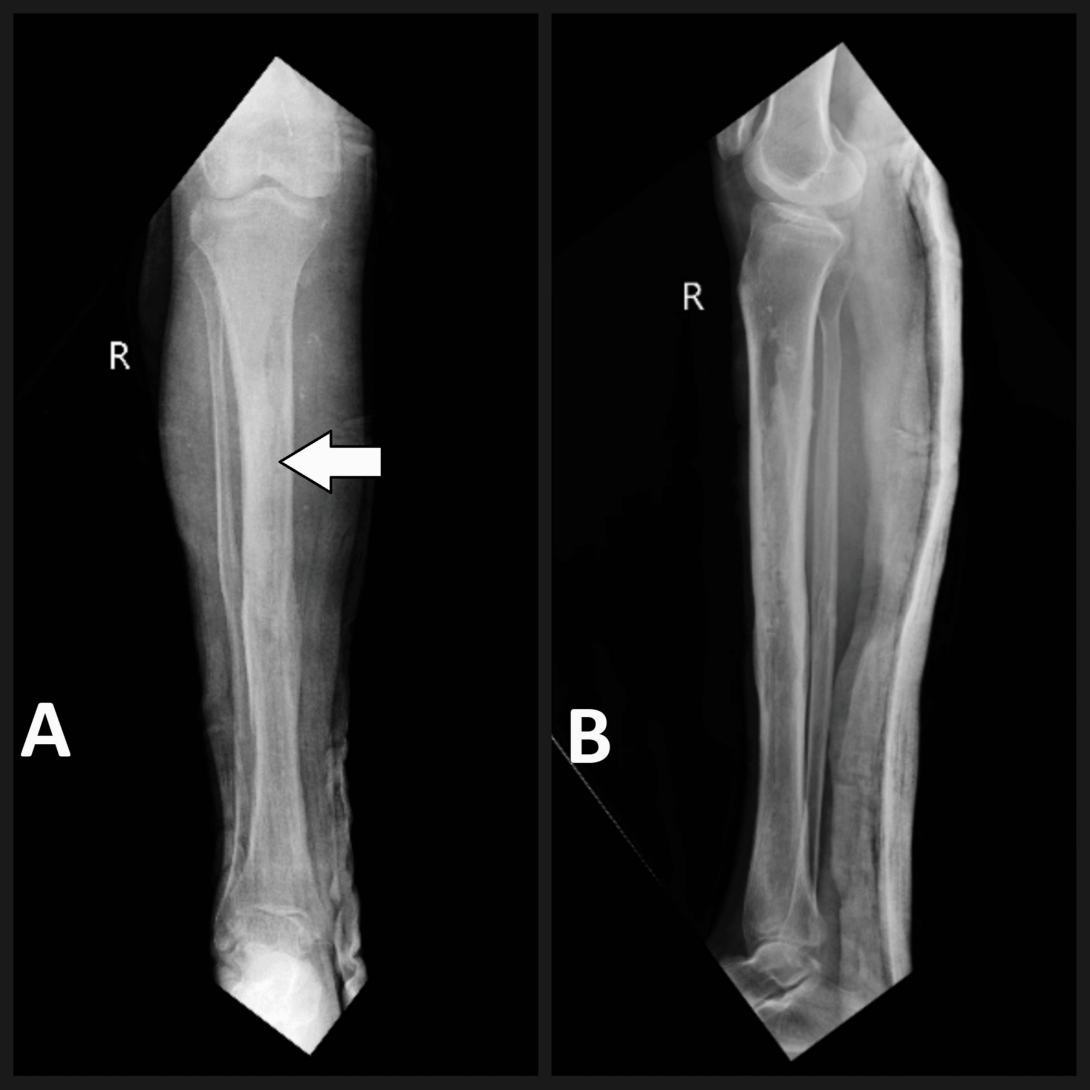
Post-operative radiograph: (A) Antero-posterior view (white arrow showing the line of vertical osteotomy) and (B) lateral view
